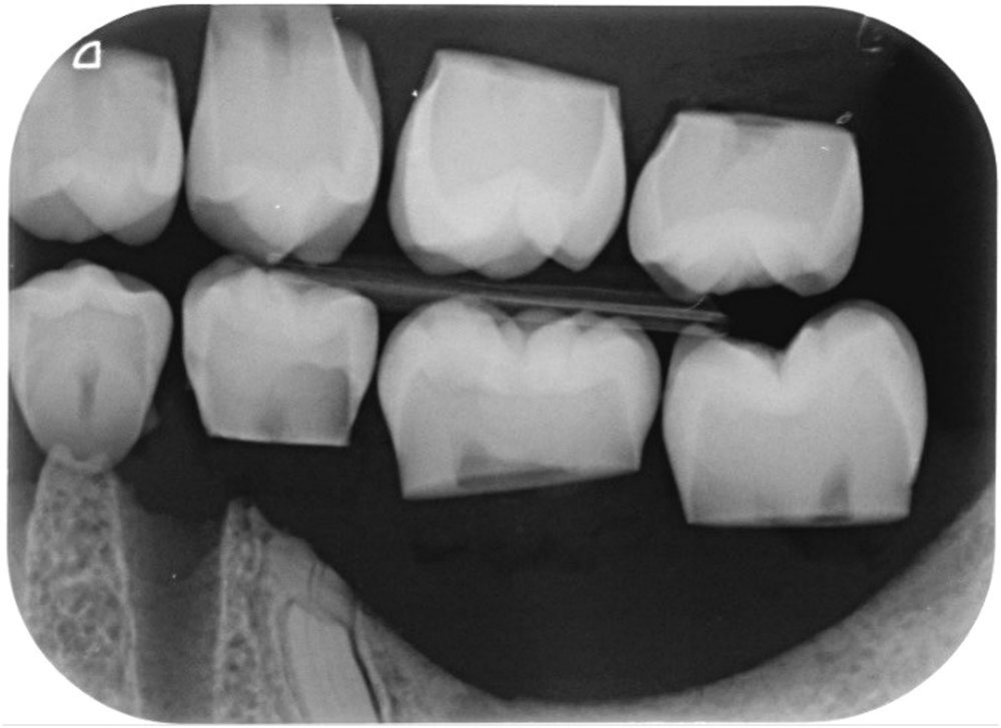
Digital bitewing radiographs of some of the sample teeth.
